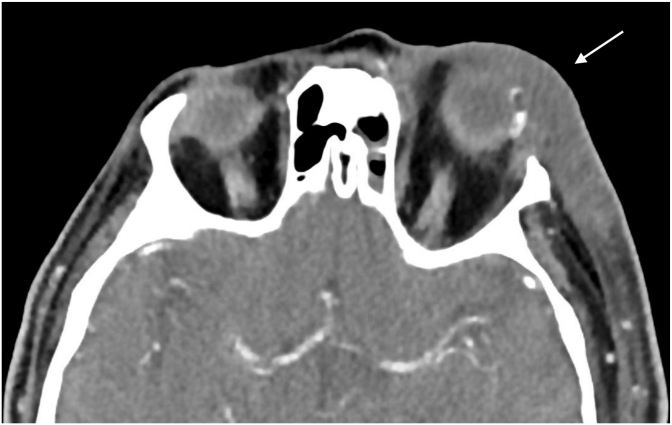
Axial contrast-enhanced CT of the orbits demonstrating marked left periorbital soft tissue swelling with fat stranding. There is evidence of exophthalmos of the left globe and a taut optic nerve.
